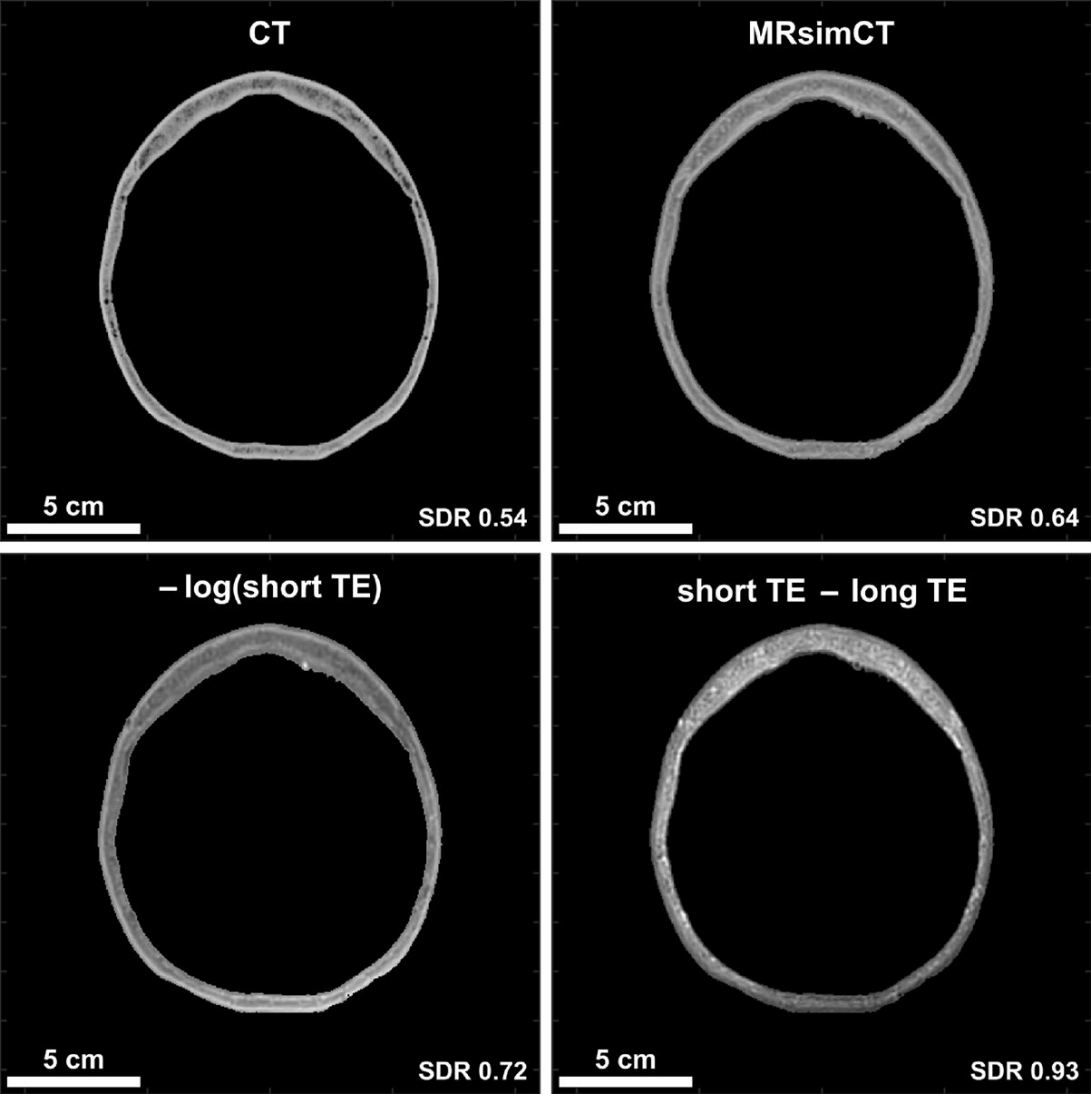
CT contrast of the skull compared to various MR contrasts (skull A). Cortical and trabecular bone contrast is clearly depicted by CT and may be preserved by two of the three MR post-processing methods. The units for MRsimCT, –log(short TE), and short TE–long TE are not the same, thus different windowing and leveling were used. Scale bars and skull density ratios (SDRs) are shown at the bottom of each image. MRsimCT was the preferred choice based on bone contrast, minimal SDR change compared to CT, minimal background signal bias, and generalizable post-processing. Therefore, it was used for the remainder of this study.
